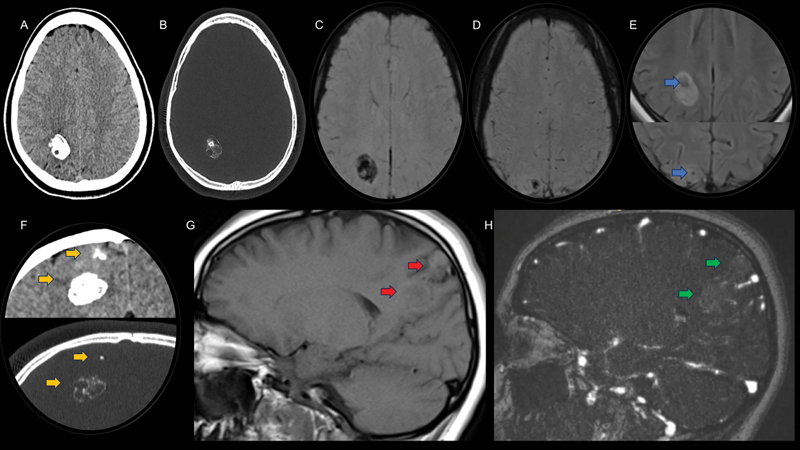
Head computed tomography (CT) scan (
